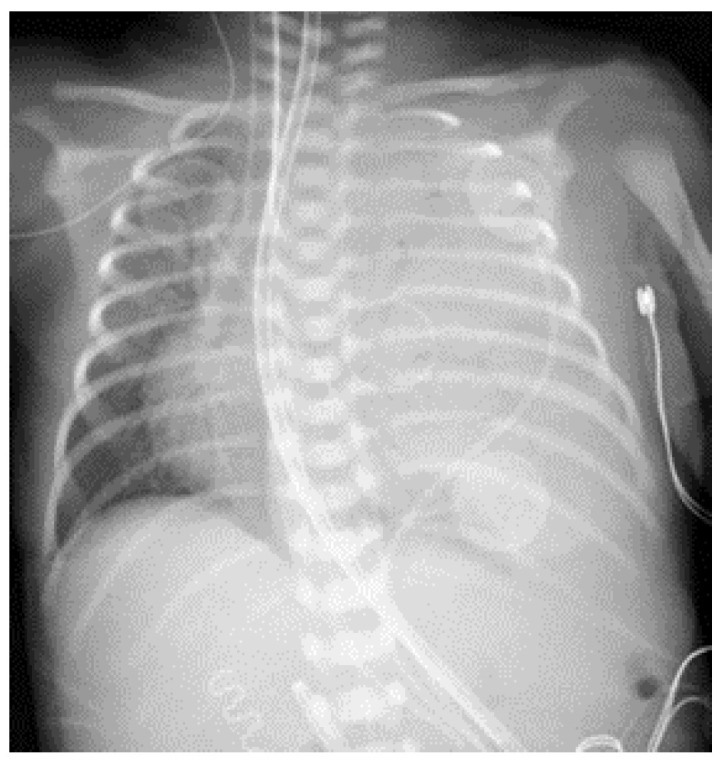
Day 1: chest x-ray showed complete opacification of the left hemithorax with mediastinal shift. Correct localization of the endotracheal tube, naso-gastric tube, and central venous access can be seen.
